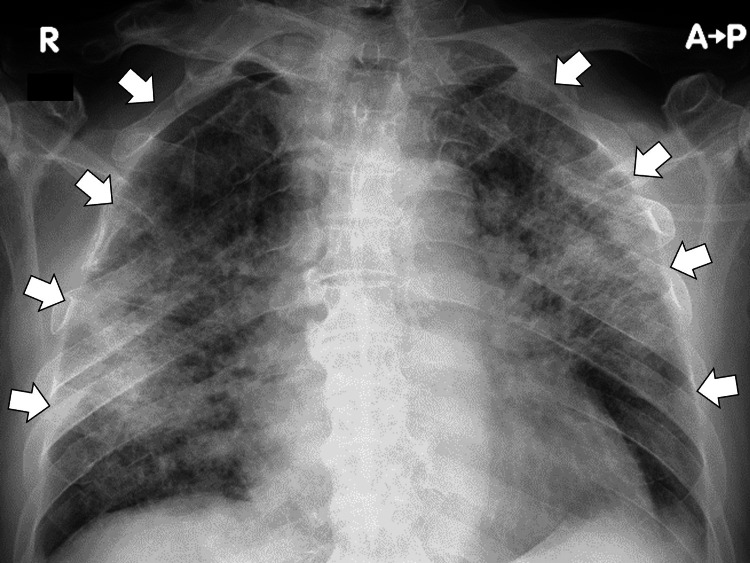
Chest X-ray revealing bilateral infiltrates (white arrows)
